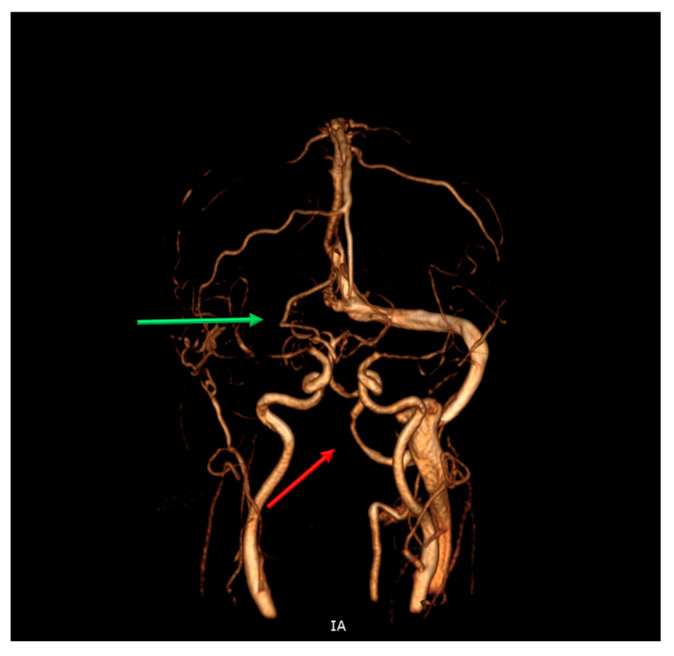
Venous MRI angiography (PHASE CONTRAST) performed after 6 months shows lack of flow in the right vertebral artery (red arrow) and in the right transverse and sigmoid sinuses (green arrow).
